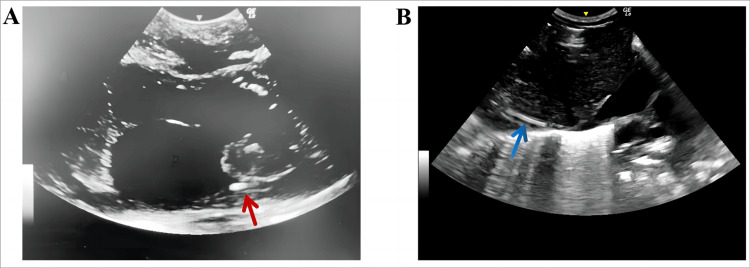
Peripherally inserted central catheter ultrasound imaging of the vena cava. (A) Superior vena cava (red arrow). (B) Inferior vena cava (blue arrow).
